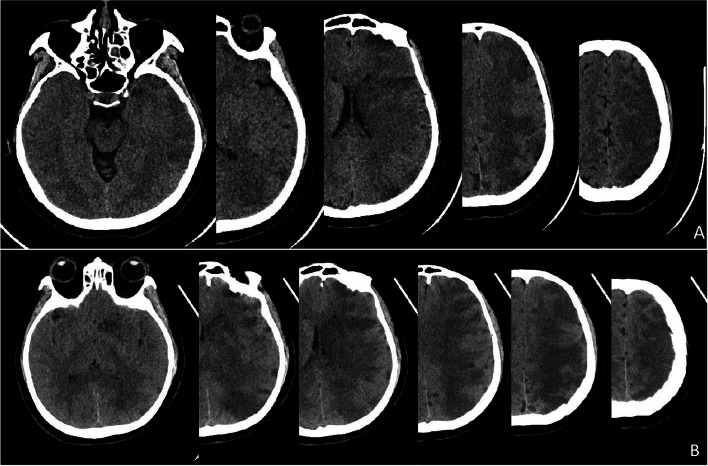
A. A repeat CT 12 h after surgery suggested a large cerebral infarct on the left side of the brain. B. A repeat CT on the 1st day after surgery suggested increased infarct edema
